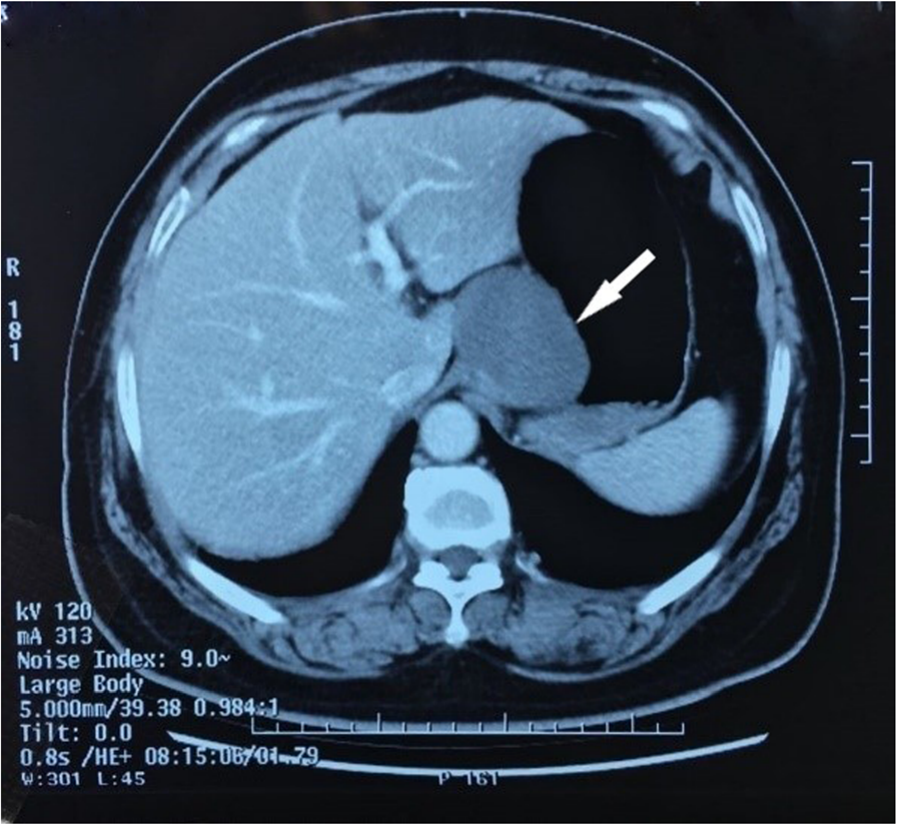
Abdominal enhanced computed tomography. The mass appeared as a quasi-circular object adjacent to the lesser curvature. Its size was approximately 6.4 × 4.9 cm, and it had a uniform density with no obvious enhancement
